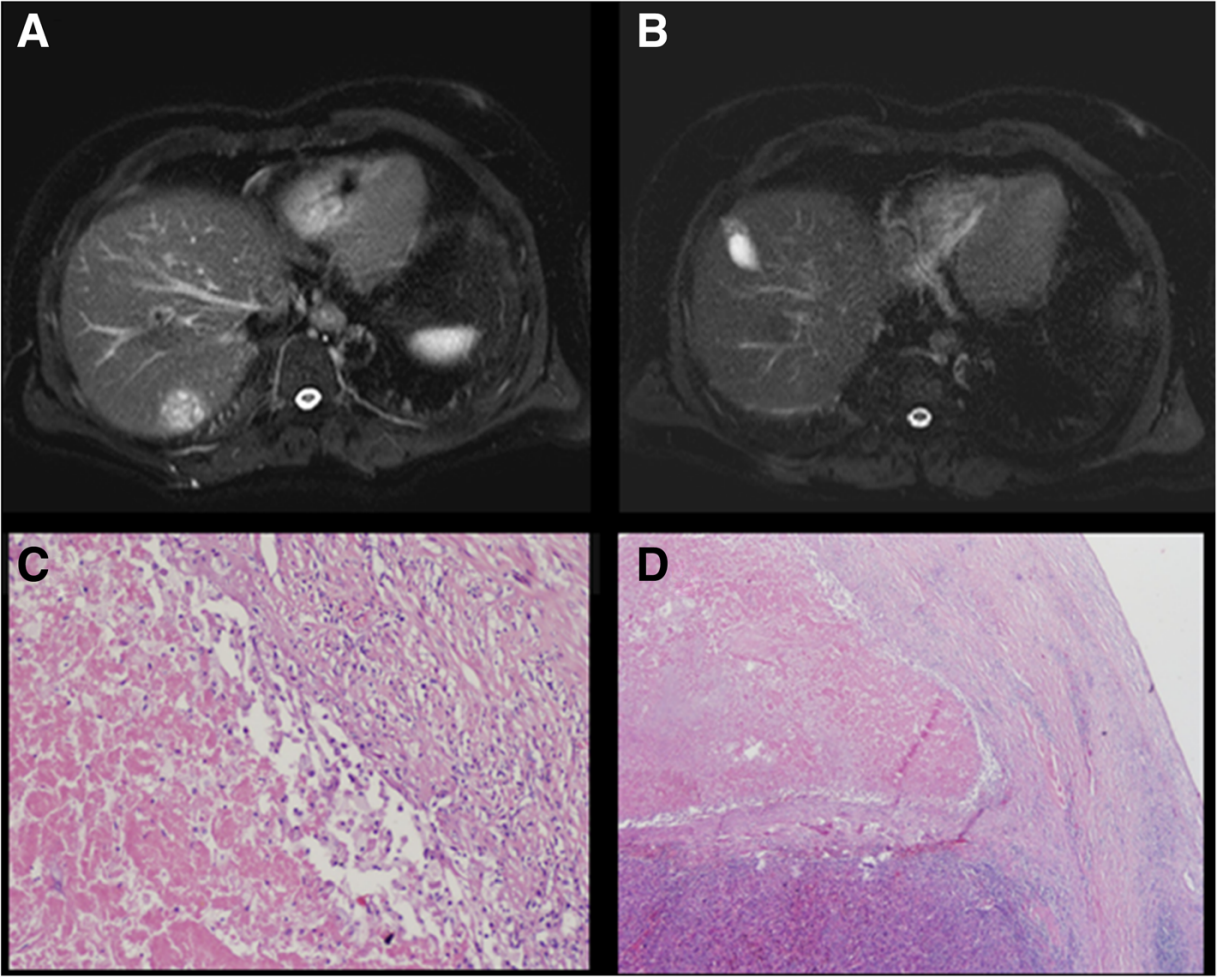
a A pre-treatment MRI scan of an HCC nodule in May 2013 (b) An MRI scan in May 2016, demonstrating a durable tumor response to SBRT (c + d) Liver explant pathology from an HCC nodule treated with SBRT, demonstrating areas for coagulative necrosis and areas of loss of tumor morphology
